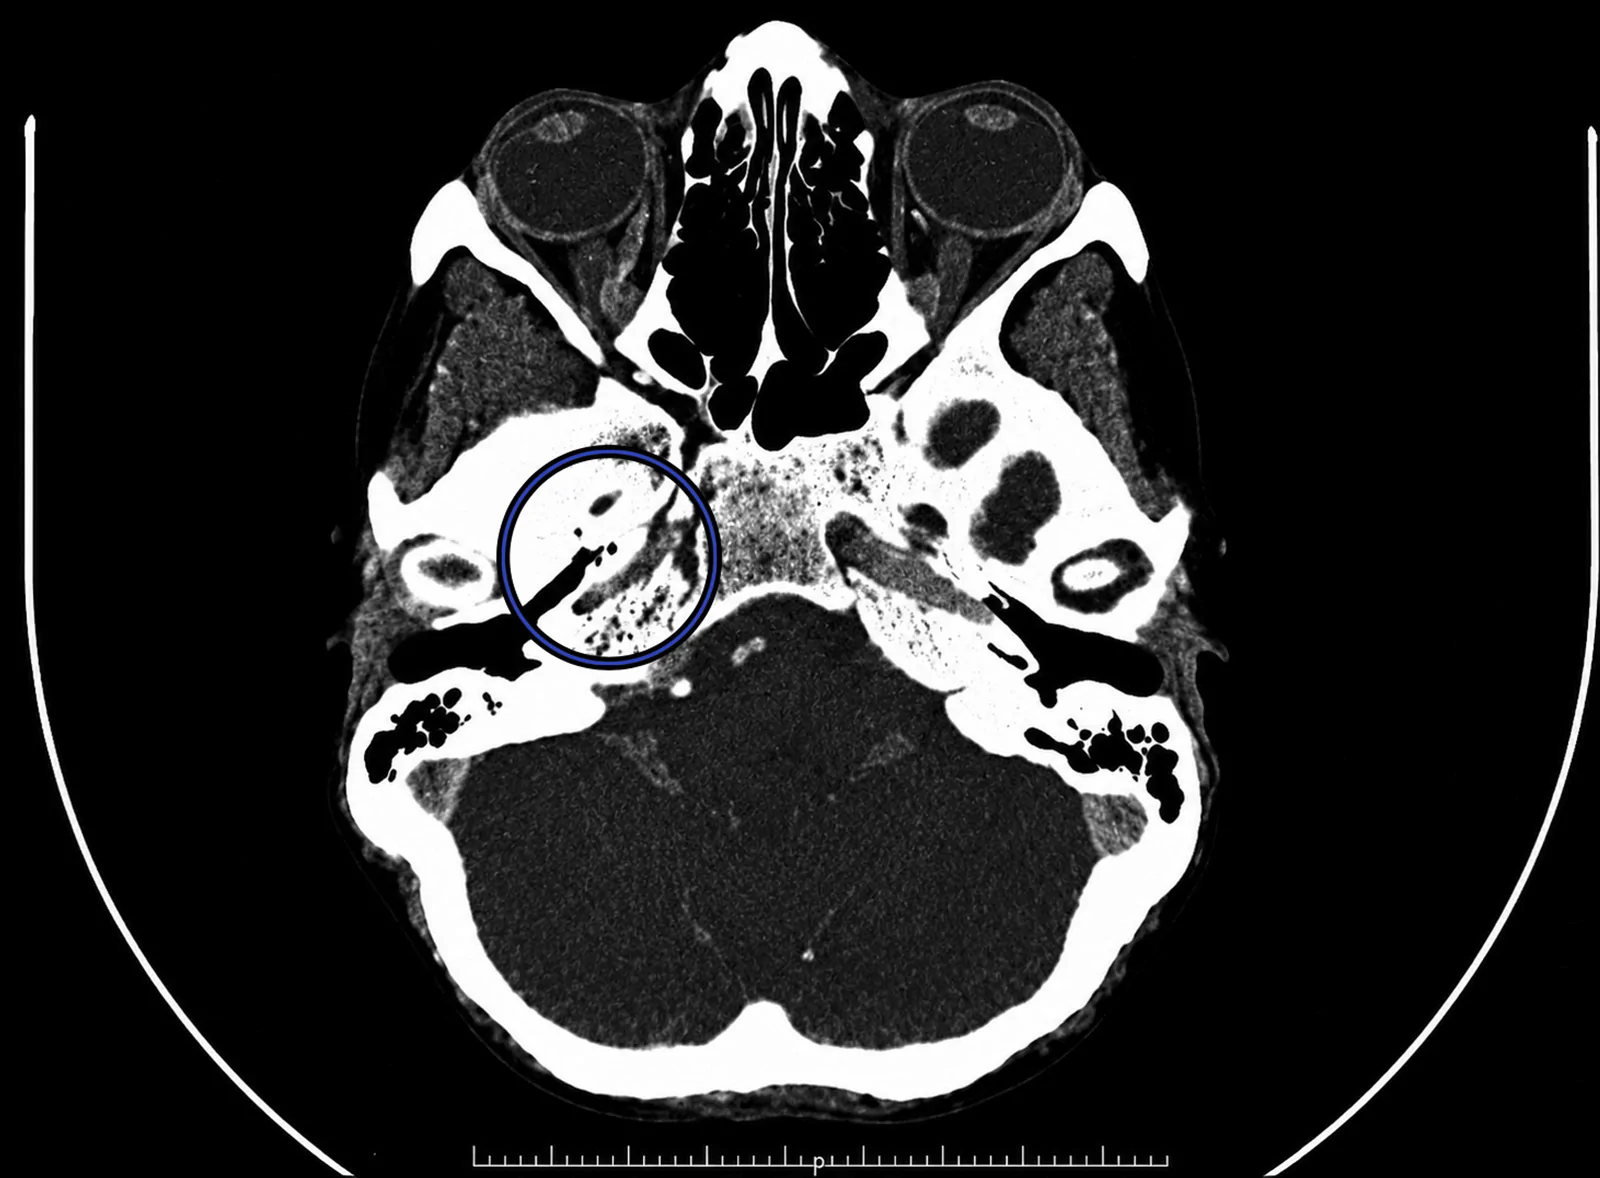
Carotid and cerebral computed tomographic angiography The right internal carotid artery diffusely narrowed (blue circle) in its intracranial course in the petrous segment.
